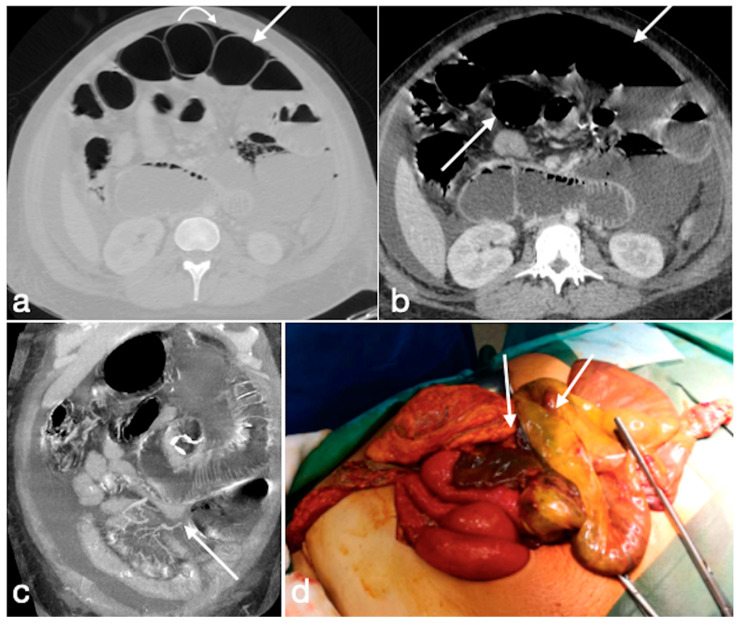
Internal hernia with bowel necrosis after cesarean delivery. A 37-year-old-female patient complaining of abdominal pain, fever, and constipation early after cesarean delivery. Note the presence of intraperitoneal free air (a, axial view, curved arrow), overdistension of a small bowel loop with thin wall, some of them with barely noticeable enhancement (a,b, straight arrows), and the convergence of bowel, mesentery, and vessels of the closed loop at the hernia orifice (c, coronal view, straight arrow). The prompt volume reduction of the lower abdomen is probably due to the delivery, which solicited the bowel herniation. The patient underwent prompt surgery confirming the CT diagnosis and leading to bowel resection due to necrosis (d, straight arrows, bowel loops in different stages of ischemia/infarction). Image courtesy of Dr. Michele Lanza, Dr. Antonio Brillantino and Dr. Maurizio Castriconi Department of Emergency Surgery, “A. Cardarelli” Hospital, Naples, Italy.
